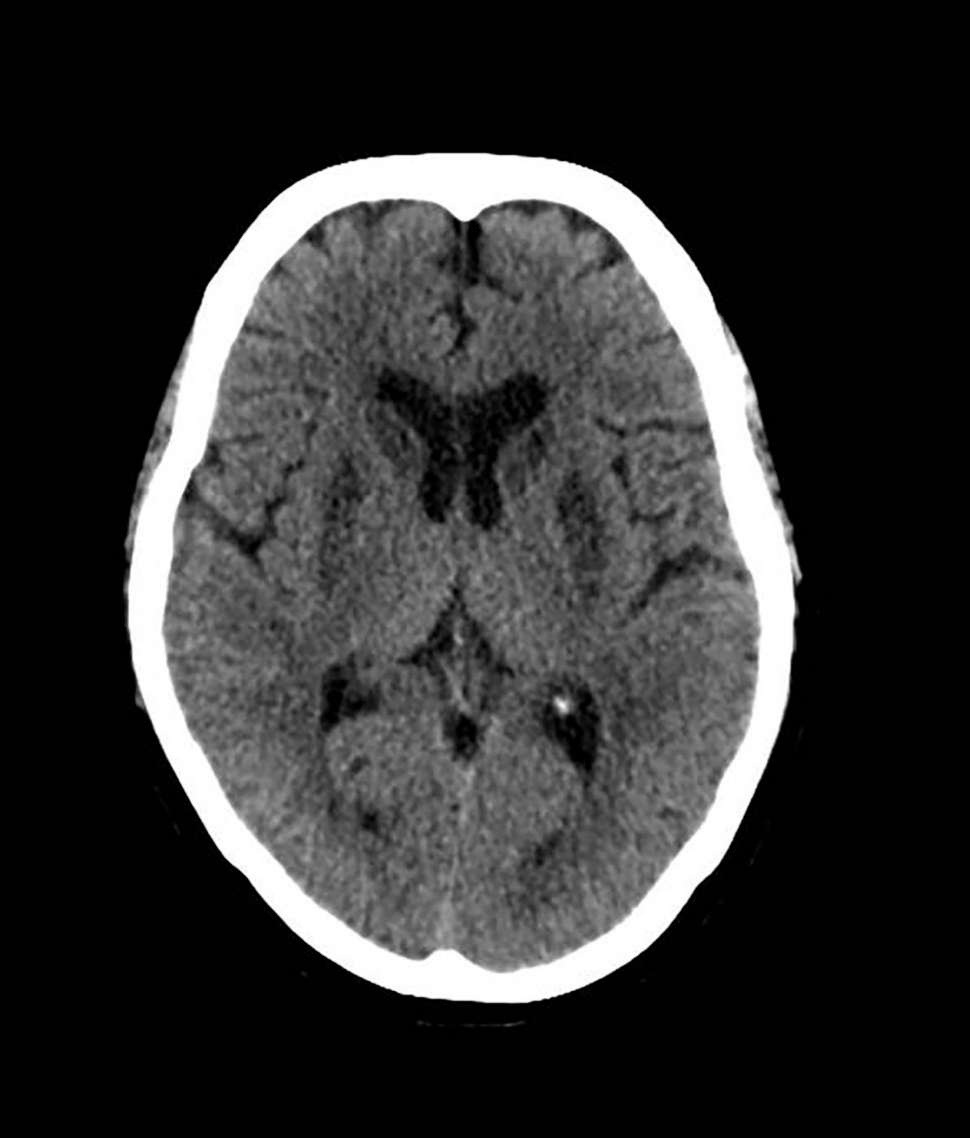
A non-contrast head CT, 6 weeks after cardiac resuscitation, showing symmetrical hypodensity of caudate nuclei and putamen
